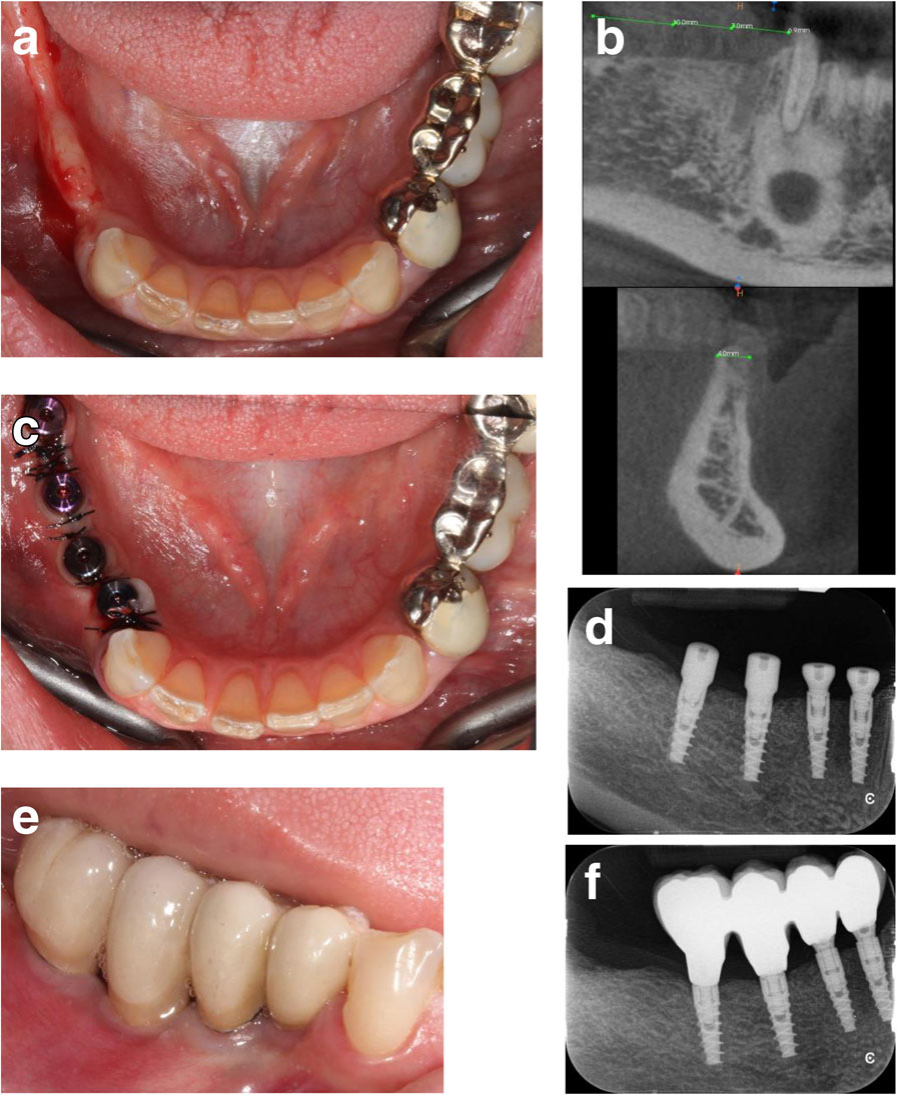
Case 1: Example of one case involved in the study. a Preoperative view of a partial edentulism in posterior mandible. b Preoperative CT scan. The width of the ridge was 4 mm. c Four narrow diameter implants were placed and left to a nonsubmerged healing. d Baseline periapical radiograph. e Buccal vieew of the final metal ceramic restoration. f Periapical radiograph at 1 year after loading
